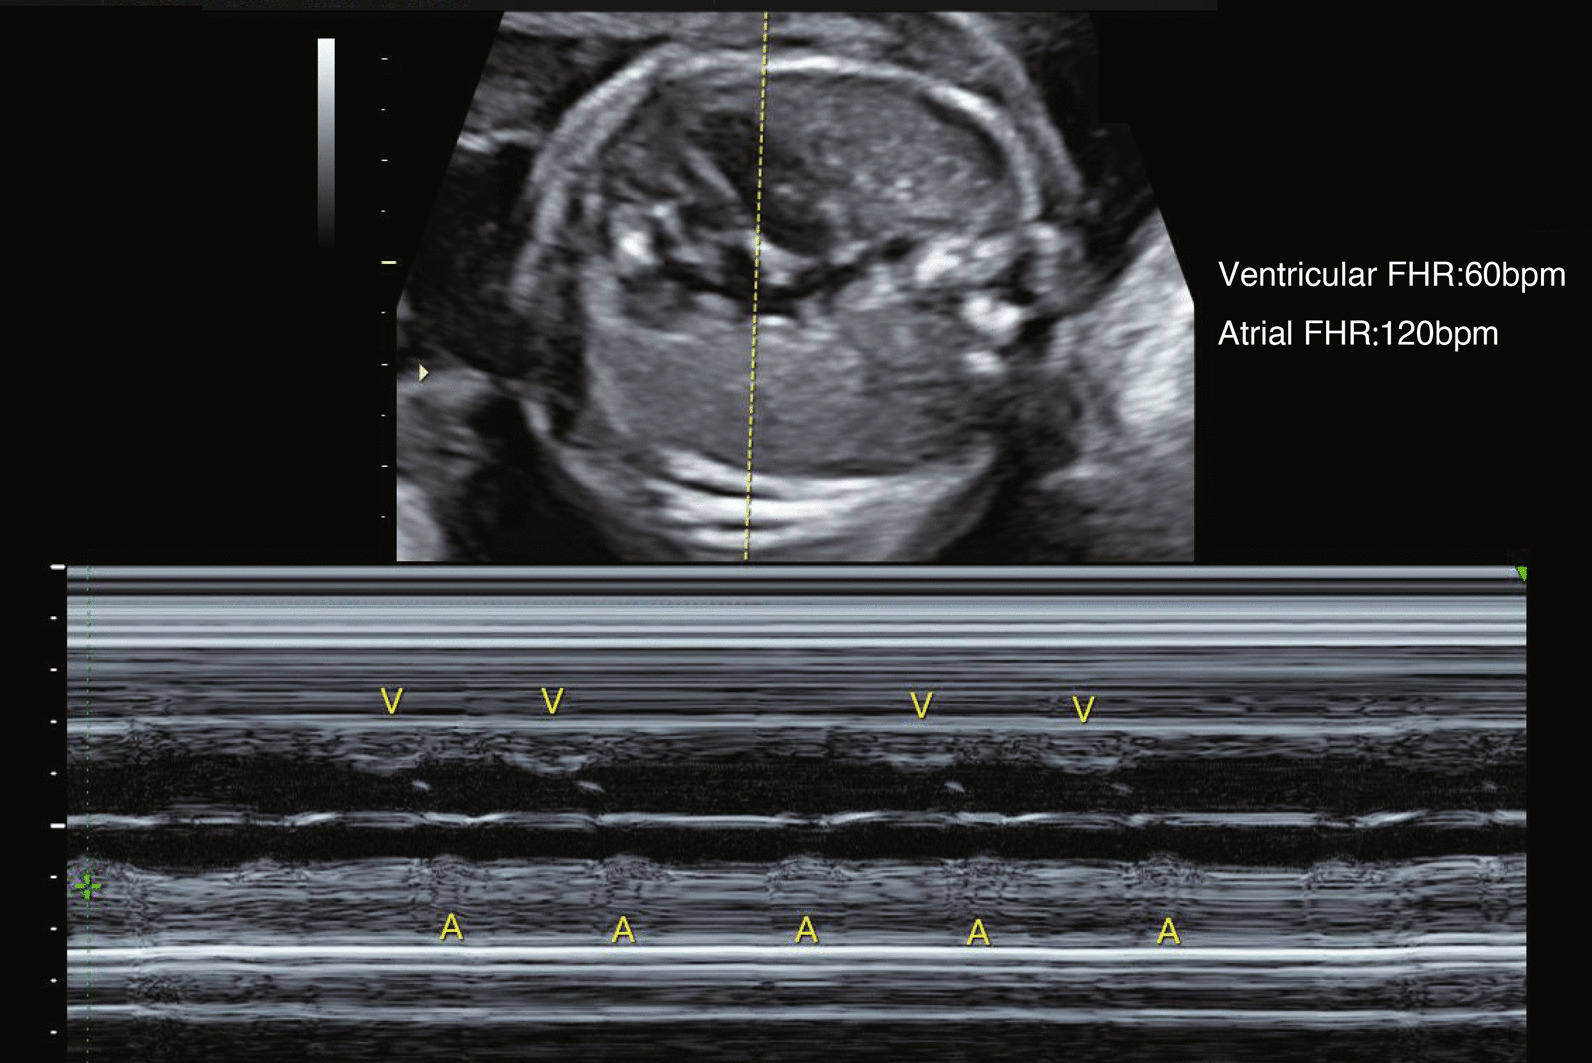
M-mode fetal ultrasound scan. Complete atrioventricular block is apparent by the atrial and ventricular wall movement recordings. *A* atrial contraction, *V* ventricular contraction, *FHR* fetal heart rate
